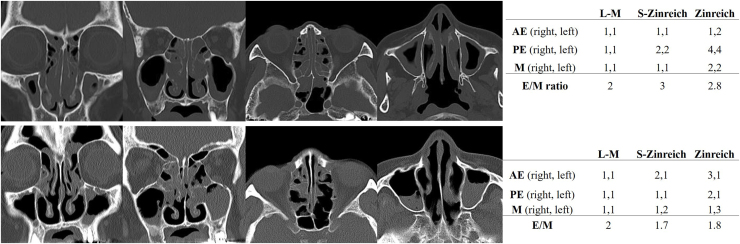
CT images showing different evaluation patterns. Axial and coronal bone window CT images. The upper case shows the presence of ethmoid-dominant shadow. The lower case shows the absence of ethmoid-dominant shadow. AE, Anterior Ethmoid Sinus; PE, Posterior Ethmoid Sinus; M, Maxillary Sinus; E/M, Ethmoid-to-Maxillary sinus ratio; L-M, Lund and Mackay scoring system; S, Simplified.
